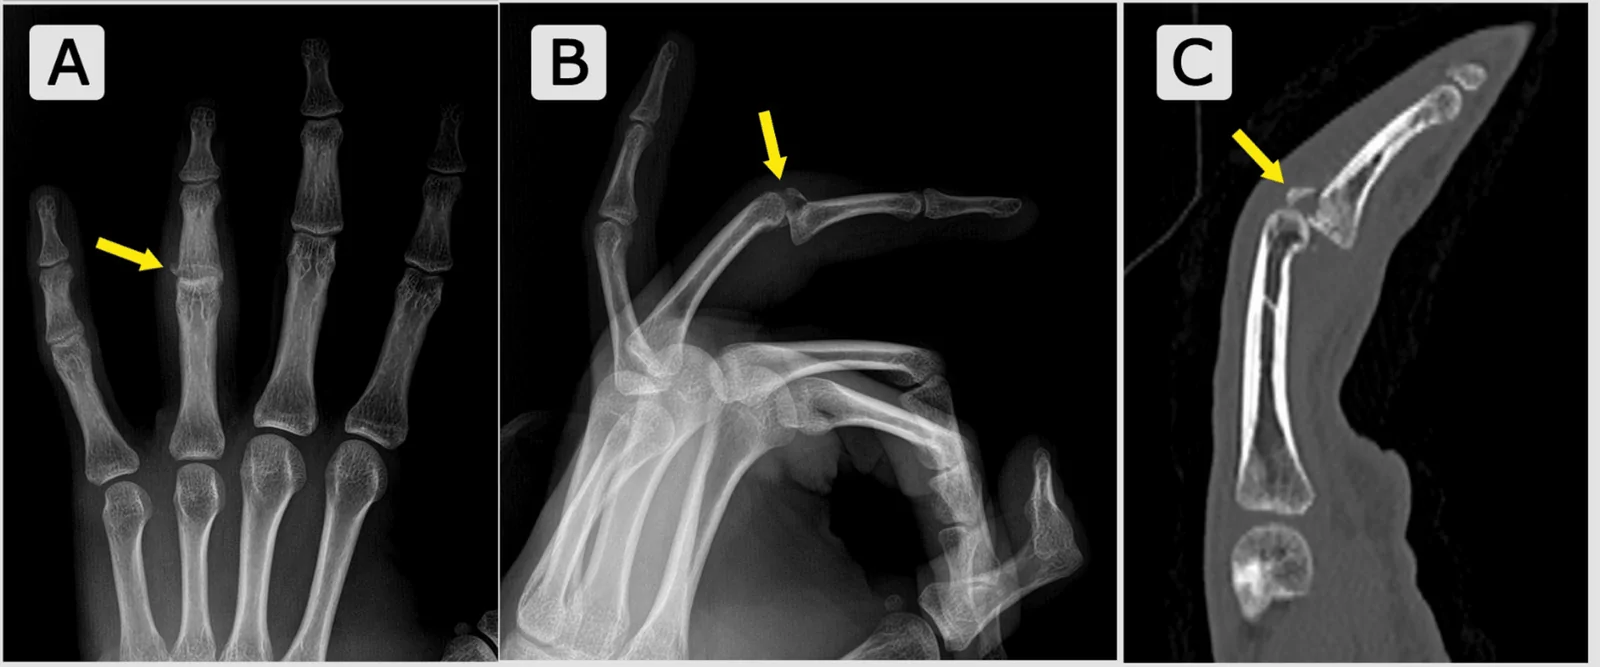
Preoperative imaging. (A) AP radiograph of the left hand demonstrating volar malalignment of the ring finger PIP joint (arrow). (B) Lateral radiograph confirming complete volar dislocation of the PIP joint (arrow). (C) Sagittal CT image demonstrating complete volar dislocation with a small dorsal rim fracture estimated to involve less than 30% of the articular surface (arrow) AP: anteroposterior, PIP: proximal interphalangeal, CT: computed tomography
